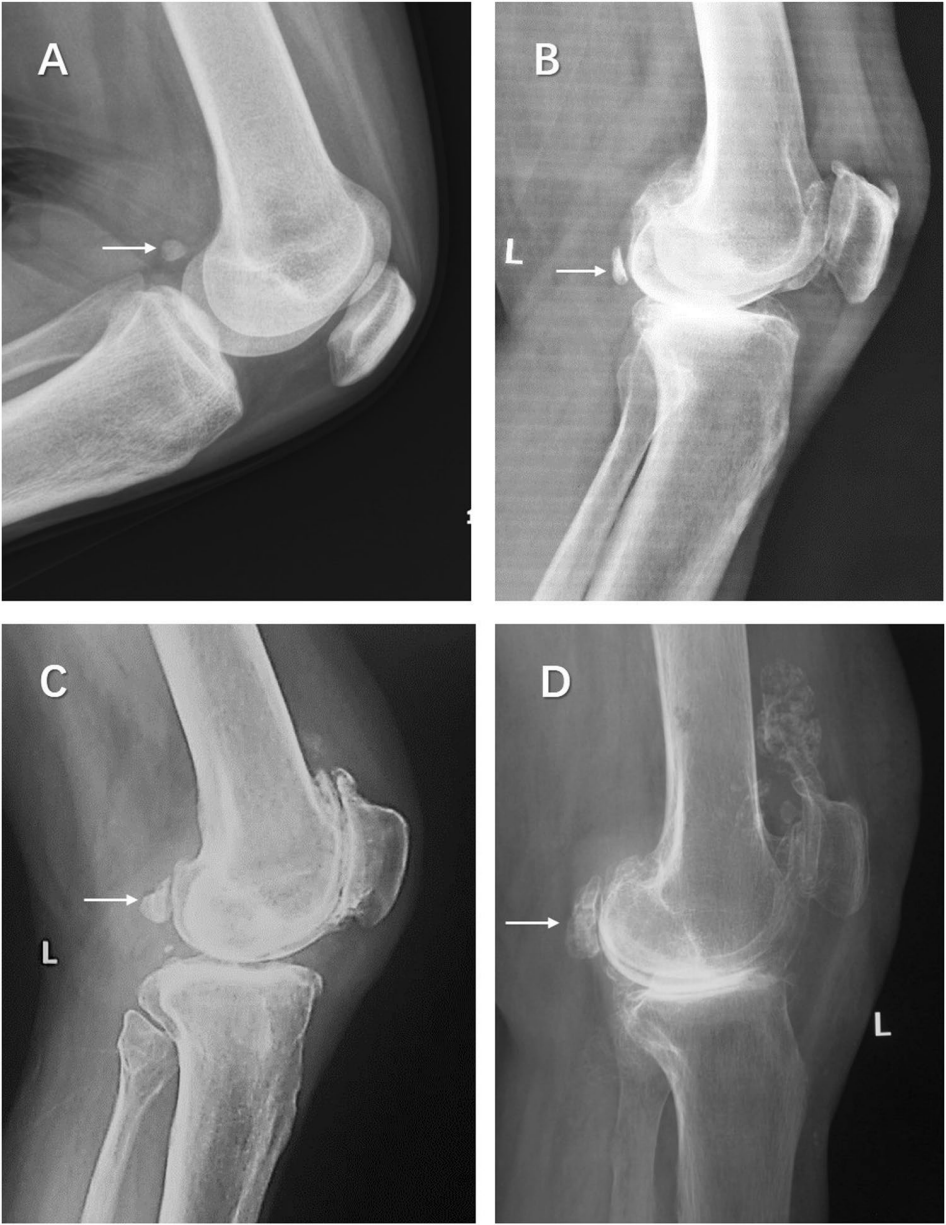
Different degenerated grades of fabellae are shown in lateral knee radiographies. (A) Grade 0, normal fabella, which is oval shaped, and anterior smooth surface is articulated with posterolateral condyle of femur; (B) Grade 1, sclerosis fabella, with anterior surface subchondral sclerosis of the fabella; (C) Grade 2, severe sclerosis of the fabella with osteophyte formation; (D) Grade 3, large fabella with marked osteophyte formation. Arrows point to the fabellae.
